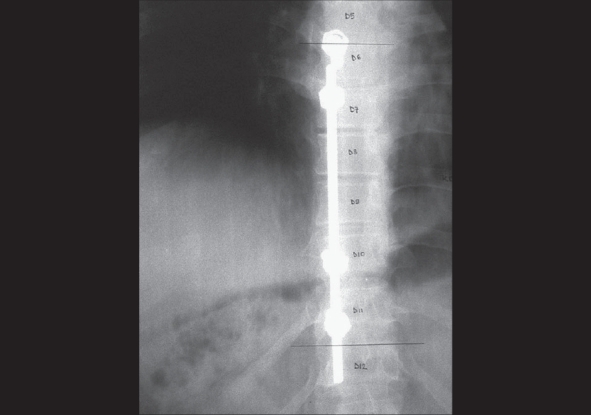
Four-year follow-up AP radiograph showing loosening of the rod from the screw at the upper end. The rod and screws were subsequently removed
